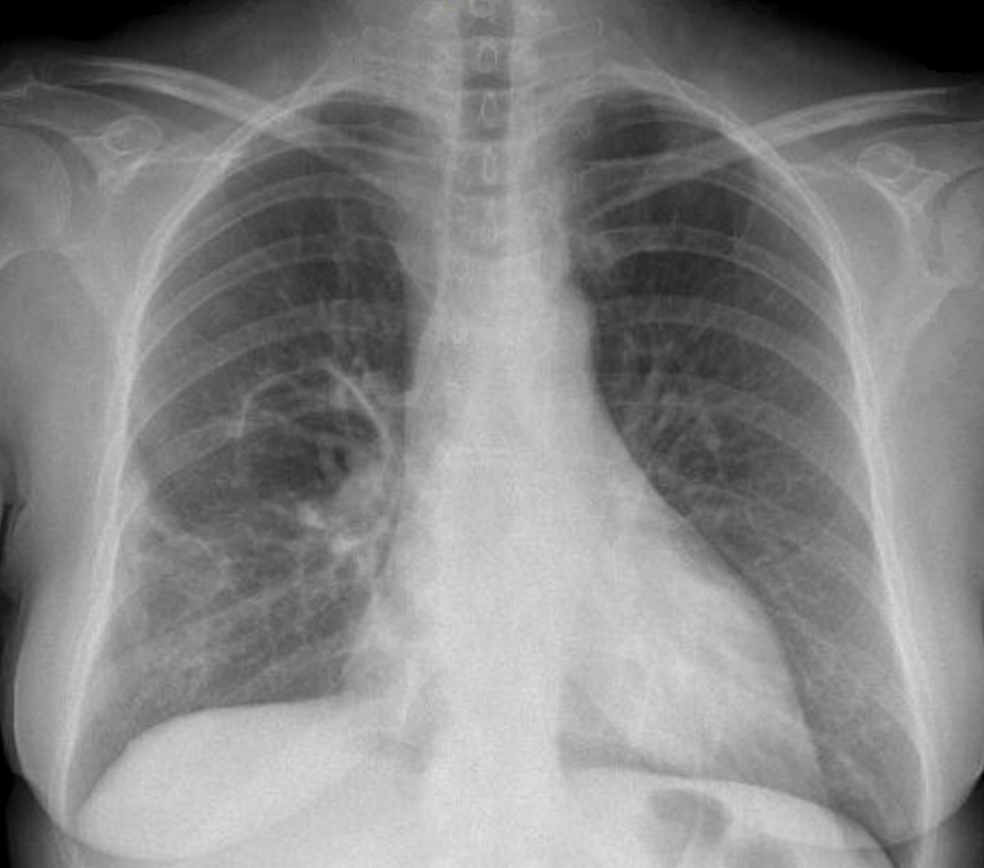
Chest x-ray done three months after initial treatment of GPA The image shows improvement in cavitary lesions when compared with prior imaging as seen in Figure 1. GPA: granulomatosis with polyangiitis
